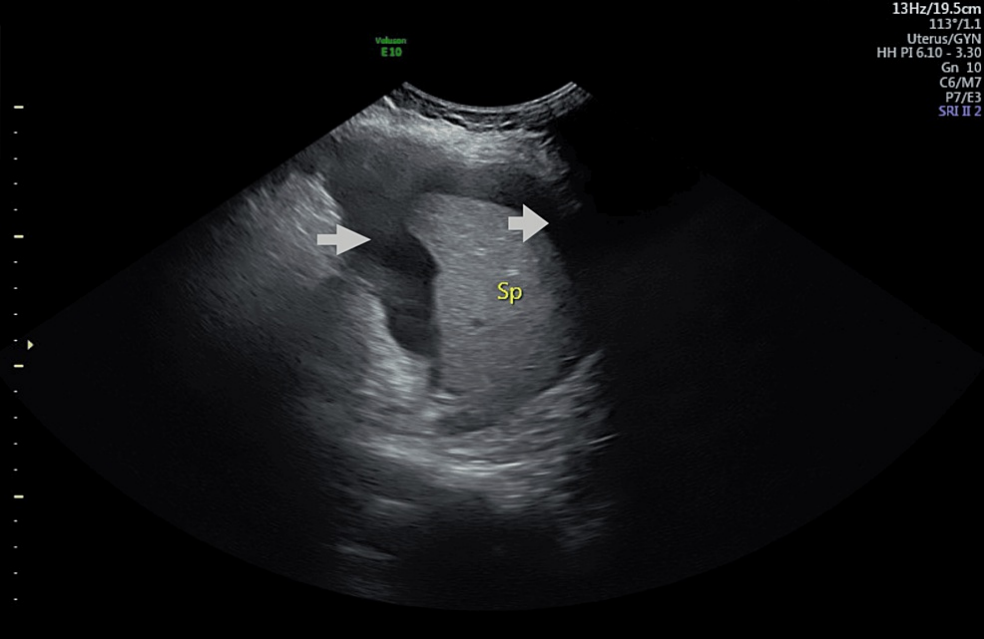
Ultrasound scan of the left upper abdomen Arrows show free fluid around the spleen. Sp: Spleen
